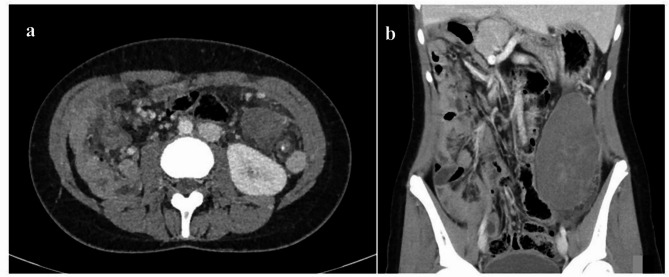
Axial (a) and coronal (b) CT scans confirm the presence of a mass and show a “whirlpool sign” involving the vascular pedicle of the twisted spleen, with a central “white dot sign”
